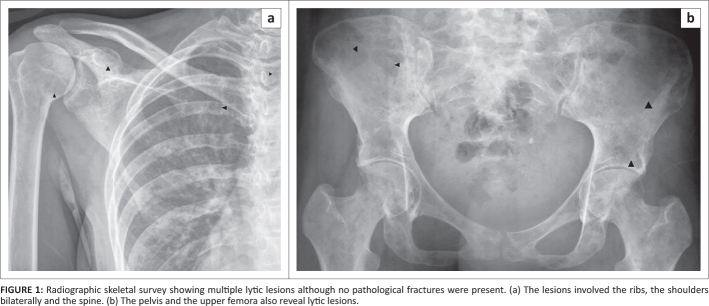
Radiographic skeletal survey showing multiple lytic lesions although no pathological
